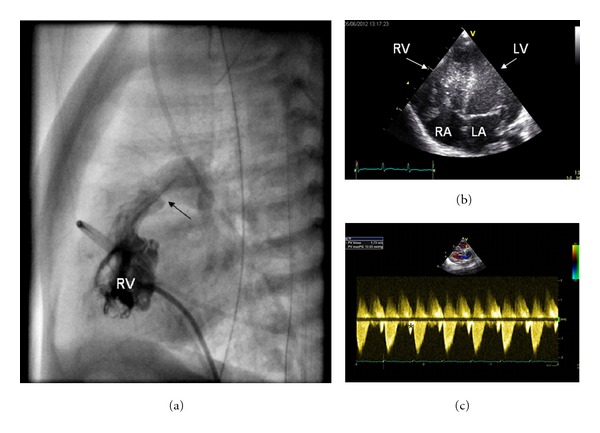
(a) Depicts the stenosis of the pulmonary valve during contrast injection into the right ventricle (RV) obtained during cardiac catheterization. Note the very small opening of the pulmonary valve (arrow). (b) Shows an apical 4-chamber view obtained with echocardiography. Note the echogenic hypertrophied trabeculae within the cavum of the RV. (c) Shows the continuous wave Doppler tracing along the main pulmonary artery with a peak gradient of 12 mmHg. Furthermore, the end diastolic forward flow over the pulmonary valve is clearly depicted (*) as a sign of restrictive RV filling. LA: left atrium, LV: left ventricle, RA: right atrium, RV: right ventricle.
